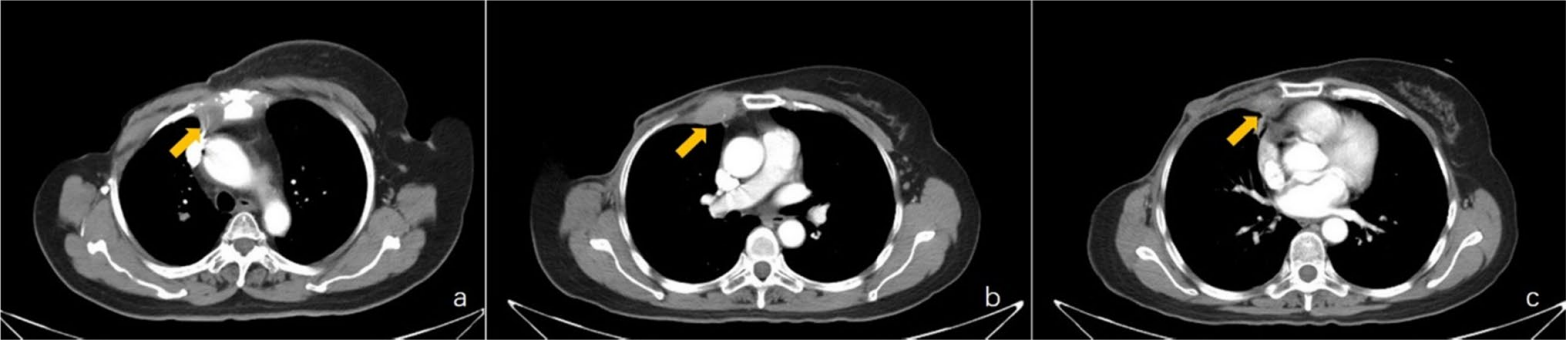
Metastatic internal mammary lymph nodes (IMLNs, yellow arrows) detected during computed tomography. A metastatic IMLN in the first intercostal space (a), a metastatic IMLN in the second intercostal space (b), and a metastatic IMLN in the third intercostal space (c)
